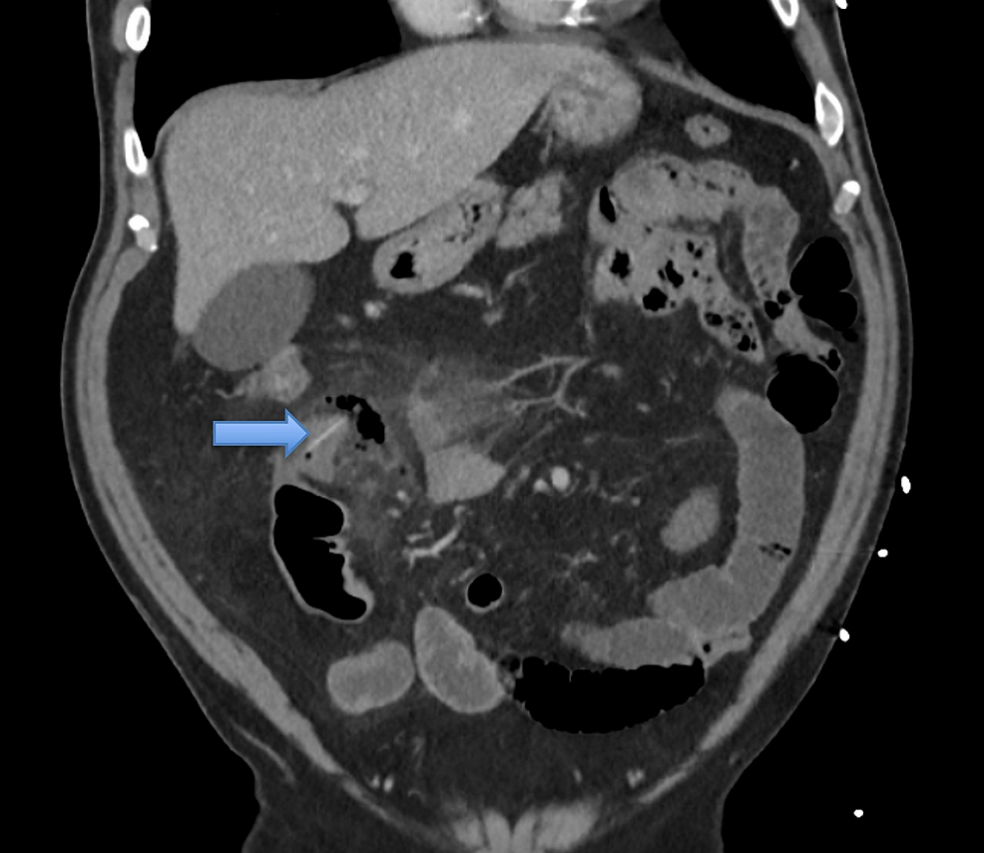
Coronal section of CT abdomen/pelvis with contrast showing perforation involving the terminal ileum. The blue arrow is pointing to the FB-BPP. The FB-BPP is not visible in the sagittal and the cross-section CT scan.
